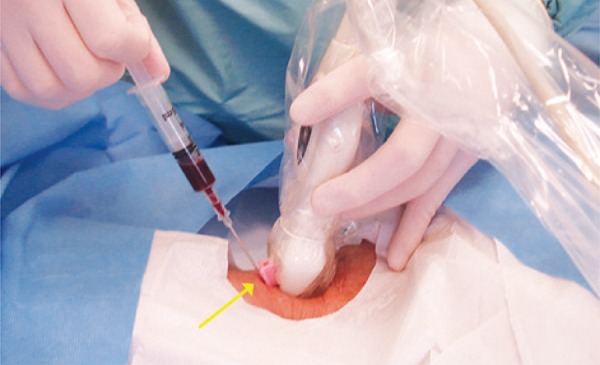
The needle guide (arrow) fixates an insertion angle of the needle in relation to ultrasound transducer
